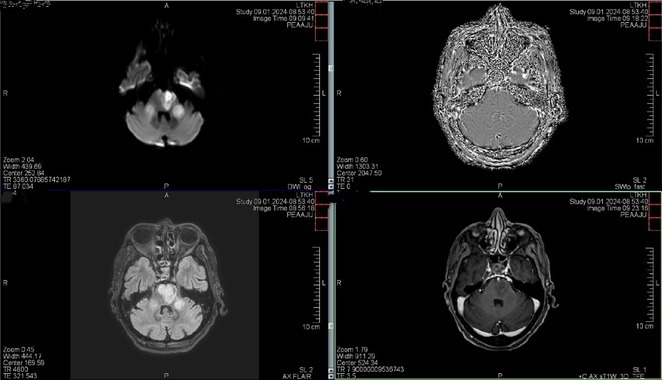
FIGURE 3 Fifth MRI scan showing ischemic lesions’ dynamic in the brainstem and cerebellar peduncles.
